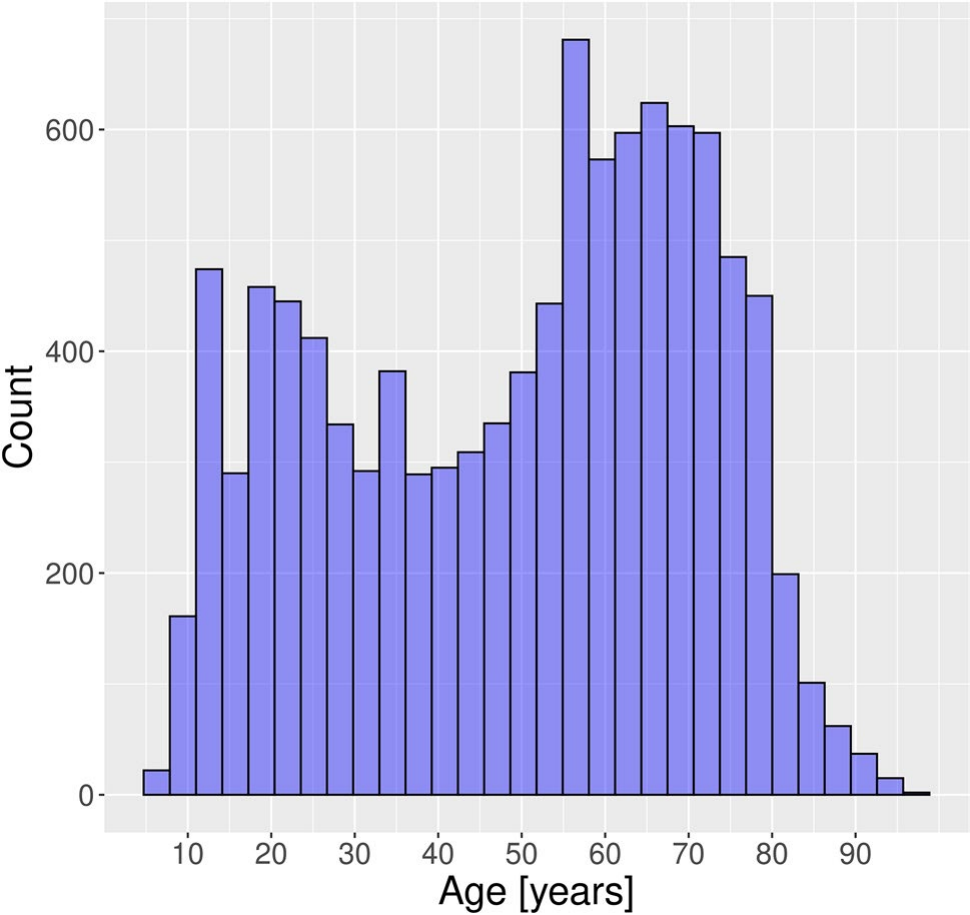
Patient age distribution of the 10,348 CBCTs
